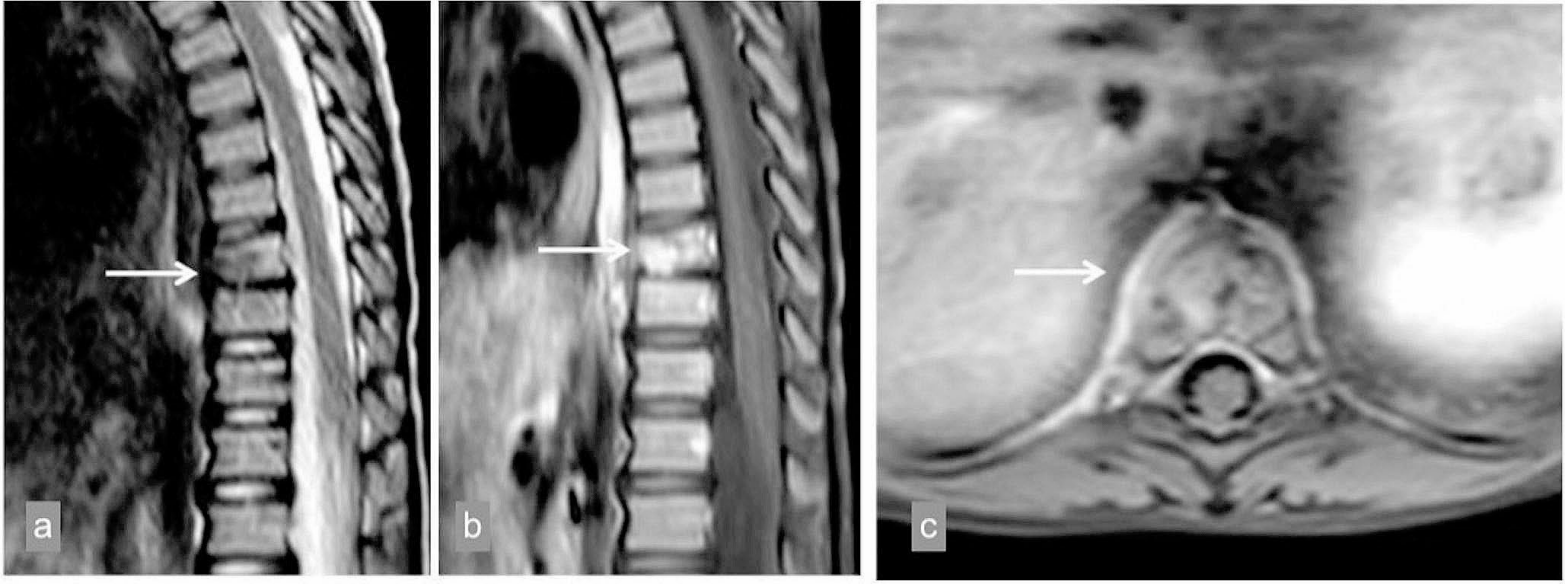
In the contrast-enhanced thoracic vertebra MRI examination taken after the treatment; Loss of height in the 9th thoracic vertebra in T2-weighted sagittal (a) images and increased contrast enhancement in the vertebra in contrast-enhanced T1-weighted sagittal (b) sections continue. However, in contrast-enhanced axial sections (c), regression was observed in the abscess formation extending to the prevertebral space (arrow)
